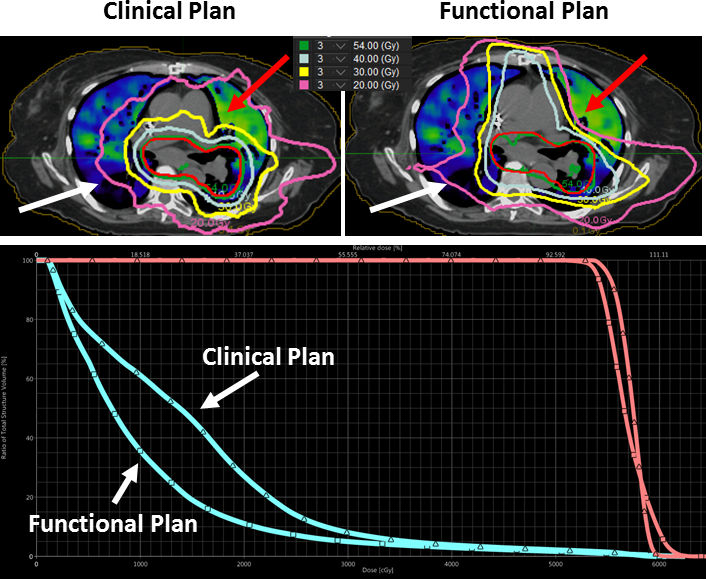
A comparison is provided between a clinical plan and the functional plan. Images on the figure display the planning CT, overlaid functional image, isodose lines, PTV (shown in red), and the DVH curves for the “functional” contour and the PTV. The red arrows highlight where the functional plan was able to spare functional portions of the lung, while the white arrows demonstrate how the functional plan deposited higher doses to nonfunctional lung (when compared with the clinical plan).
